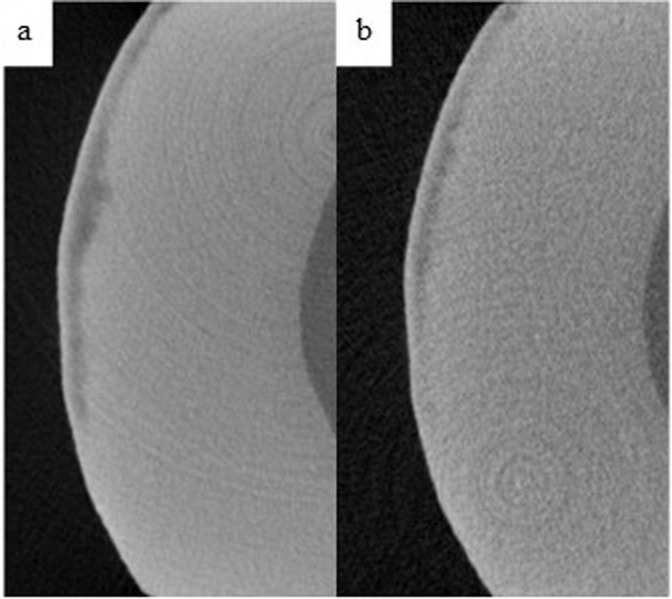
Remineralization of etched enamel MIH lesions with 1450 ppm NaF toothpaste. Representative µ-CT images of etched enamel MIH lesions, before (a) and after (b) in-situ remineralization.
